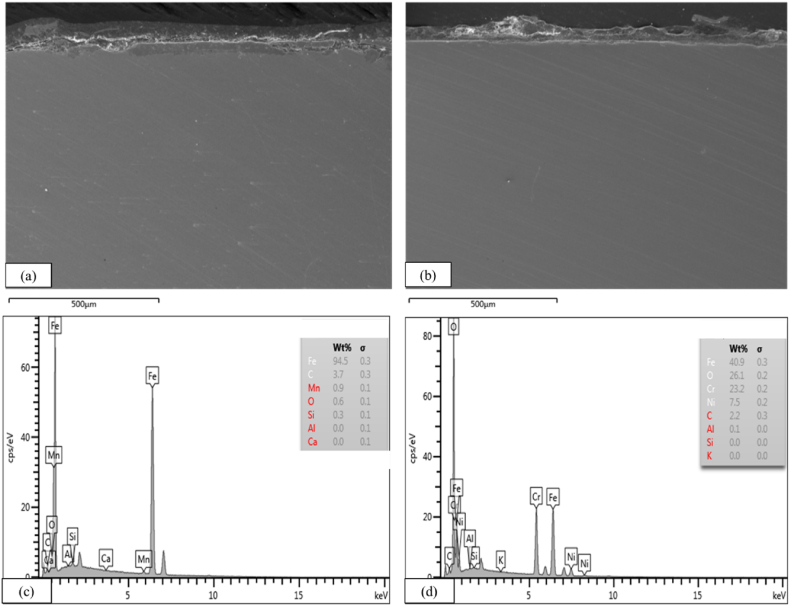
FE-SEM test photos of a) WT, b) CTA, EDS of c) WT, d) CTA.
